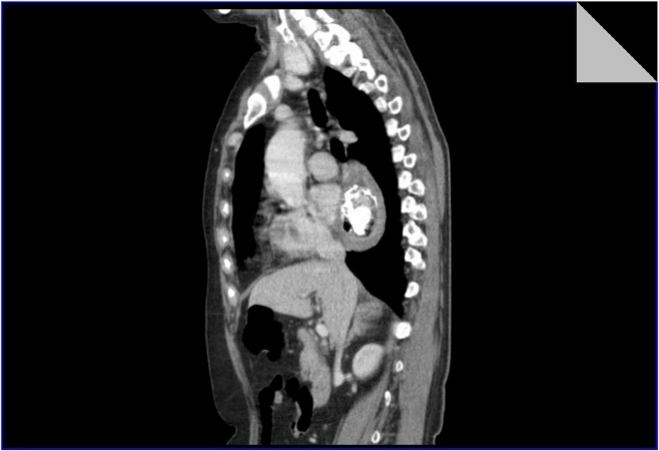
CT of the thorax excluded a pedunculated tumor, describing an intra-luminal calcified esophageal mass
